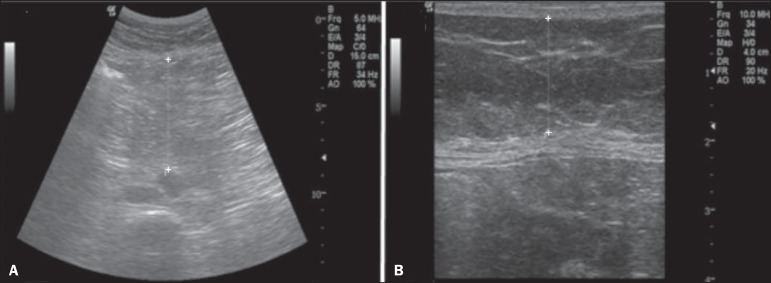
Abdominal ultrasound demonstrating the measurement methods.
